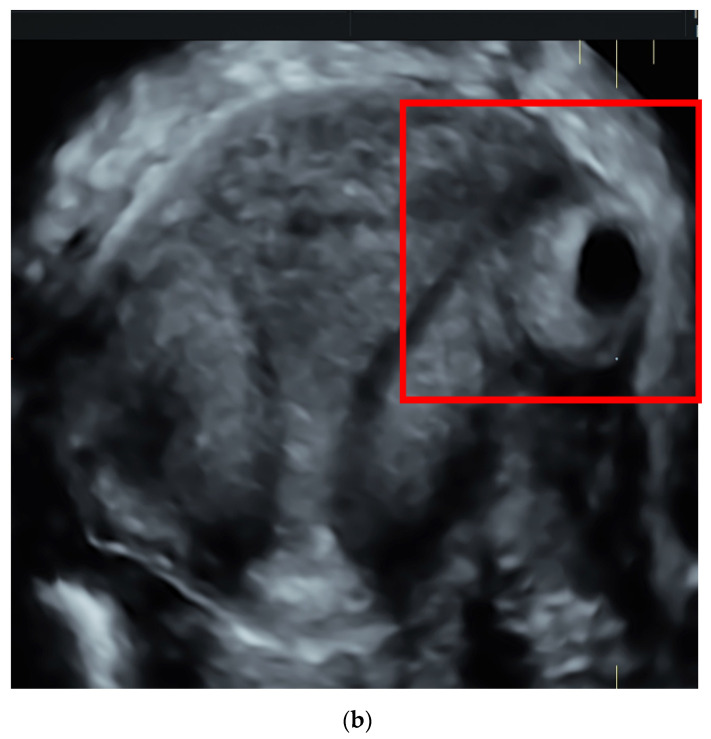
Ultrasound findings of interstitial pregnancy (a) A 3-D ultrasonography: an empty uterine cavity with a gestational sac located > 1 cm from the sideward portion of the uterine cavity; the continuation of myometrial mantle around the ectopic sac is clearly viable. (b) A 2D-view of the interstitial pregnancy surrounded by a myometrial layer of less than 5 mm.
